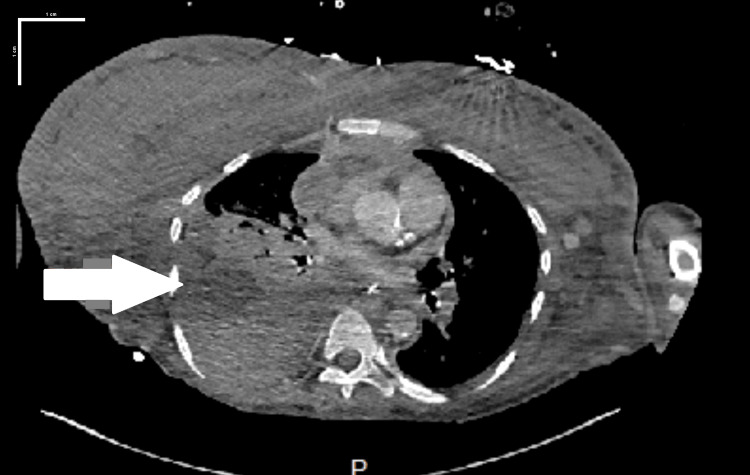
Axial section computed tomography angiography (scale bar, 1 cm) showing left-sided hemothorax.
